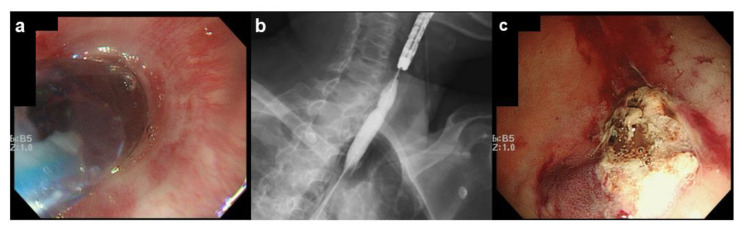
Endoscopic balloon dilation to facilitate thermal therapy at the bleeding point. (a) Endoscopic image during dilation; (b) radiograph during dilation; (c) endoscopic image after thermal therapy. The visible vessel has been cauterized, leading to complete hemostasis.
